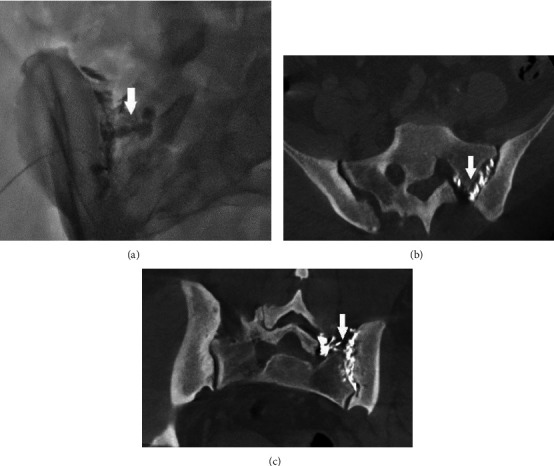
(a): Contrast spread from sacroiliac joint to S1 neuroforamen on an oblique view on the fluoroscopic image. (b): 3D-CBCT image, axial view of the sacroiliac iliac joint. Contrast spread in the sacroiliac joint and S1 neuroforamen. (c): 3D-CBCT image, coronal view of sacroiliac joint. Contrast spread in the sacroiliac joint and S1 neuroforamen (arrow indicating contrast spread into the S1 neuroforamen).
